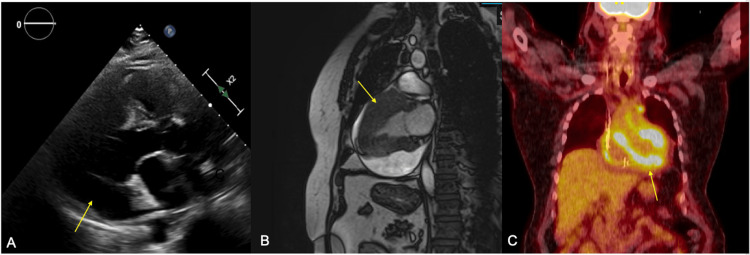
Imaging results (A) Echocardiogram demonstrating a moderate posterior pericardial effusion (free space of ~17 mm) marked with an arrow. Ejection Fraction of 60-65%, visually estimated with moderate concentric left ventricular hypertrophy (interventricular septal diameter of 1.4 cm), indeterminate diastolic function (Left atrial volume index of 19 ml/m^2^, Tricuspid regurgitant jet 2.1 m/s, E/e’ ratio 27, lateral e’ 4.5 and medial e’ 4.7). (B) Cardiac MRI demonstrating 20 mm thickness in the anterior septum, 30 mm mid-anterior wall (marked with an arrow), and inferior wall of 11 mm. Right ventricular walls also thickened. (C) PET CT scan with regional FDG uptake in the more basal portions of the anterior, inferior and lateral walls of the left ventricle, consistent with infiltrative cardiomyopathy, myocarditis or an inflammatory process. There was also para-aortic lymph node uptake.
